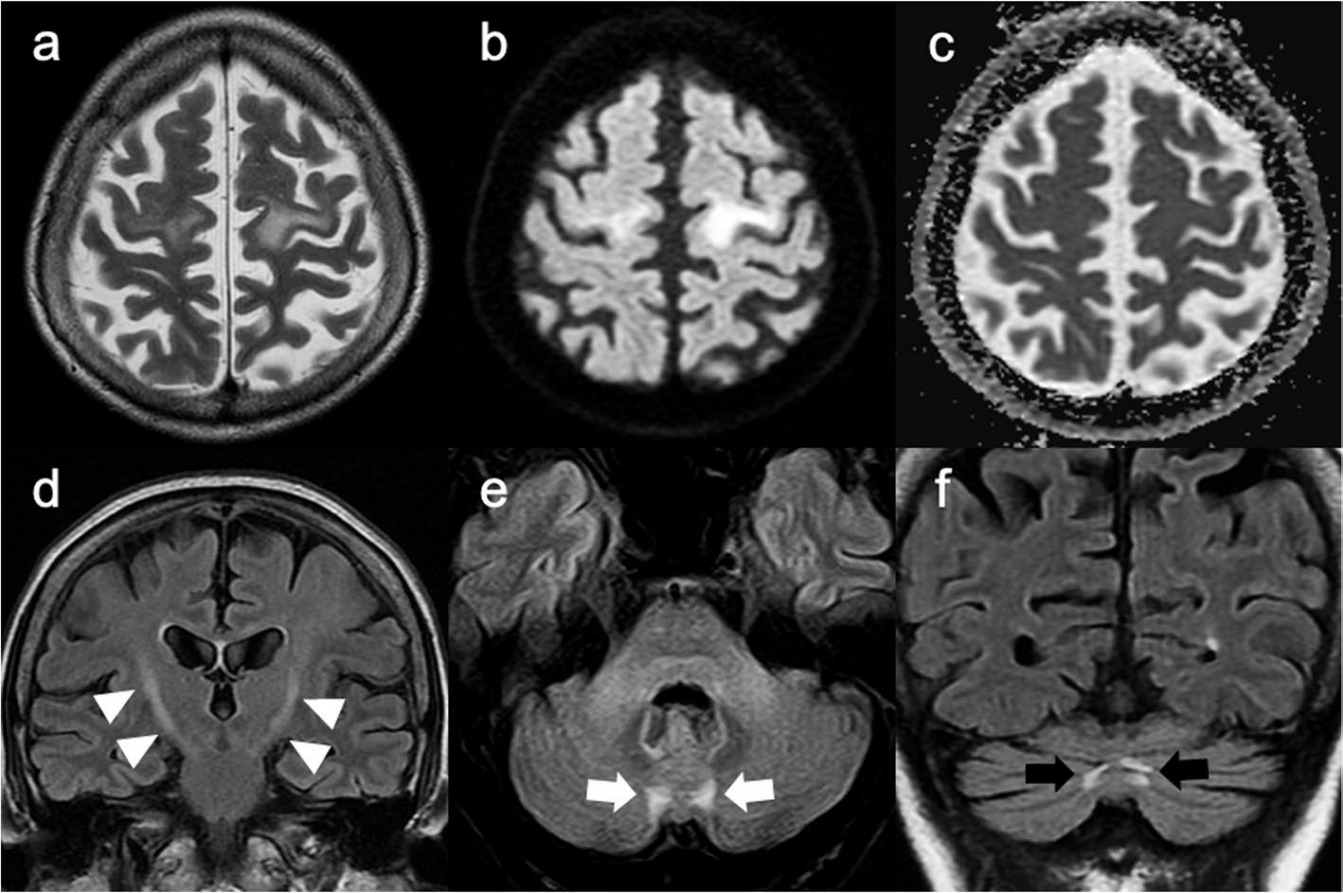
Brain magnetic resonance imaging (MRI) at the age of 60. Brain MRI shows bilateral subcortical high-intensity signals in the precentral gyri on T2-weighted (a) and diffusion-weighted (b) images. These subcortical legions were isointense on apparent coefficient mapping (c). Abnormal high-intensity signals along the corticospinal tracts are observed bilaterally on FLAIR image (d, white arrowheads). High signal intensities in the medial part of the cerebellar hemisphere right beside the vermis (paravermal lesions) are also seen bilaterally on axial (e, white arrows) and coronal (f, black arrows) FLAIR images
